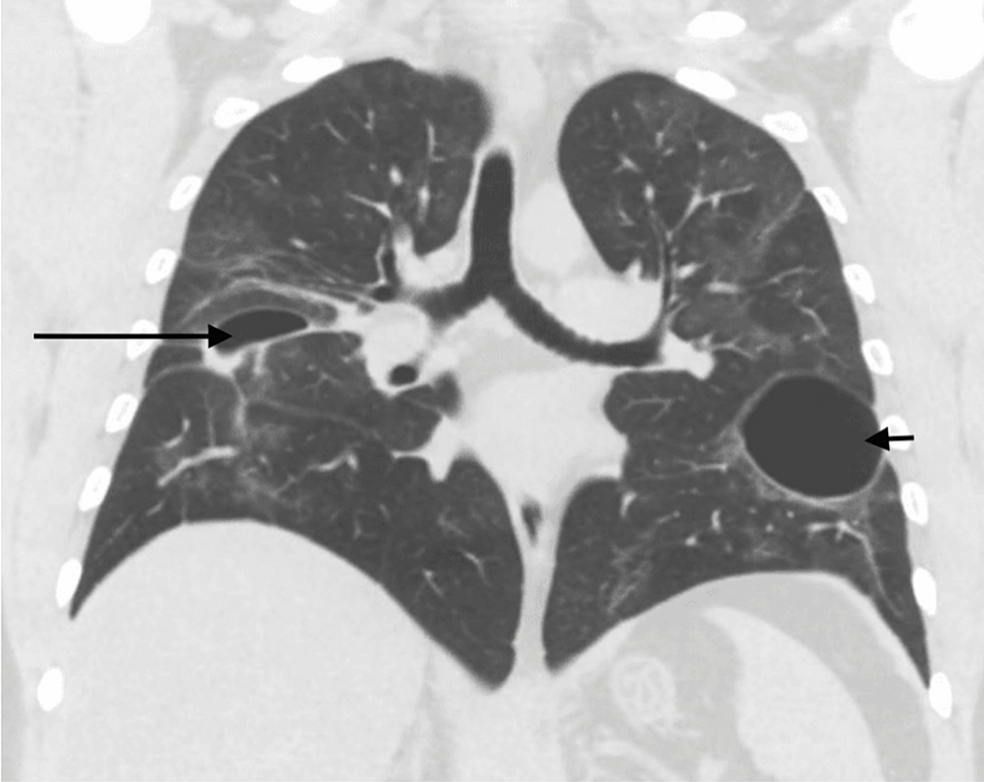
CT showing right lower lobe bulla (long arrow), which significantly decreased in size compared to the prior exam, with fluid and debris in the dependent portion and also showing new left lung bullae (short arrow)
